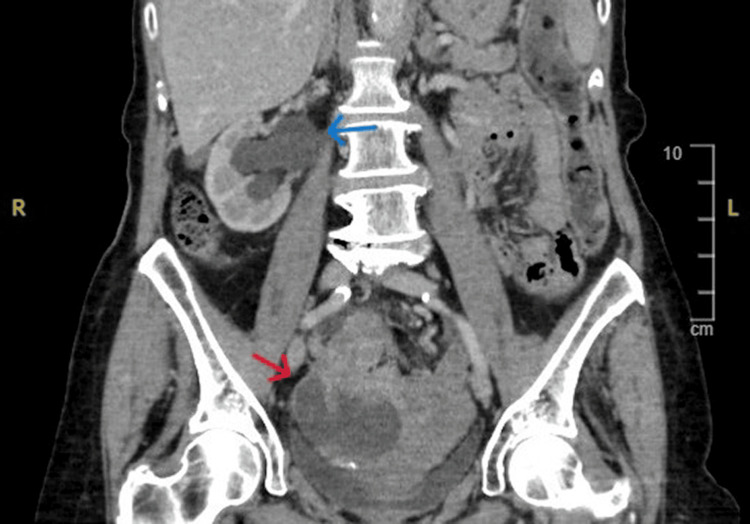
Abdomen and pelvis CT scan demonstrating right-side hydronephrosis (the blue arrow) and pelvic collection (the red arrow). CT: computed tomography.
